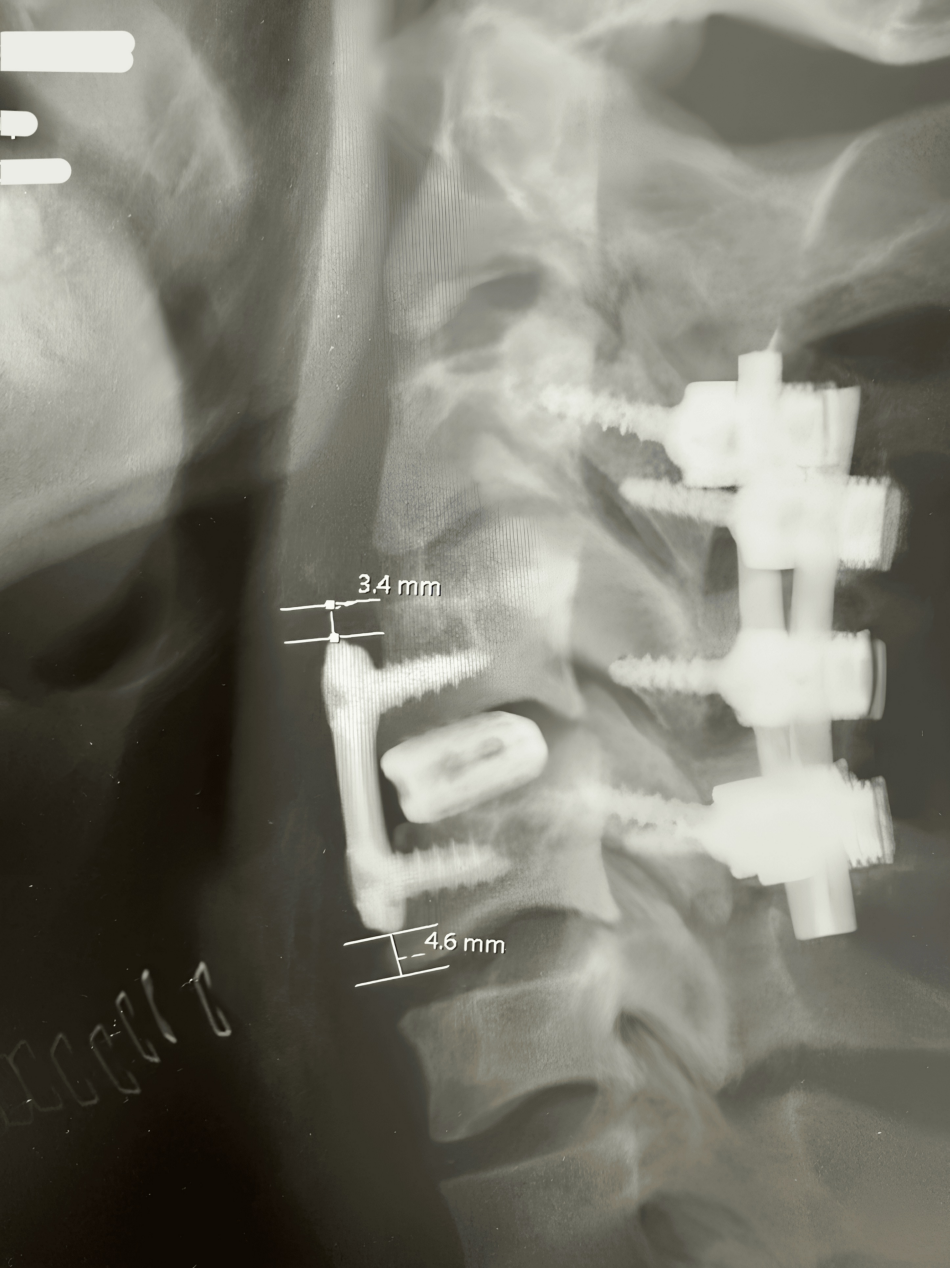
CT scan of the cervical spine after ACDF showing the method of calculating the PDD CT: computed tomography, ACDF: anterior cervical discectomy and fusion, PDD: plate-to-disc distance
